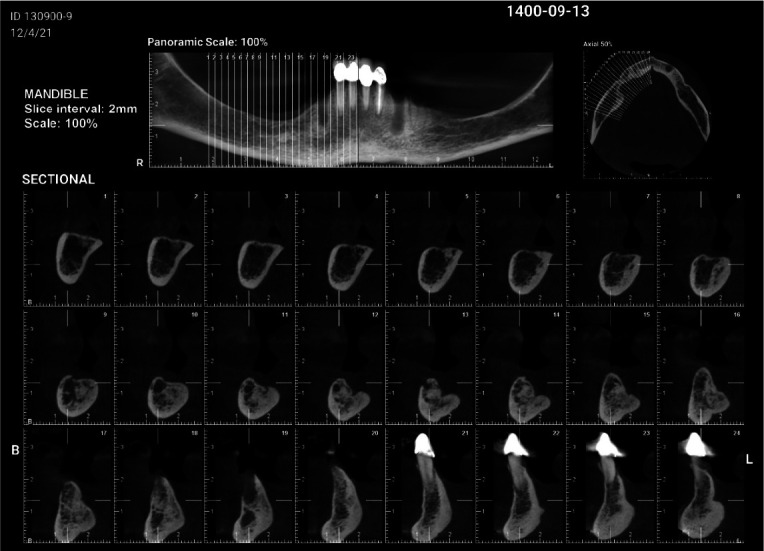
2-year cone beam computed tomography (CBCT) follow-up shows healing at the site of the lesion
